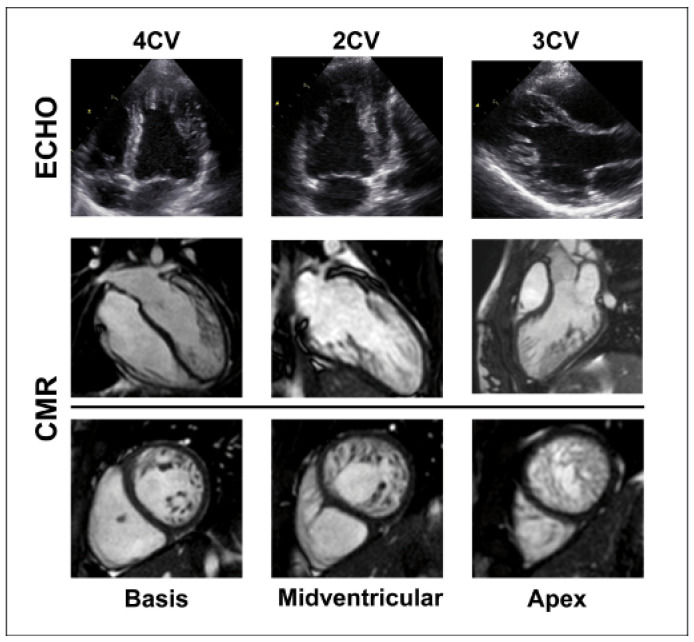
Echocardiography and cardiovascular magnetic resonance (CMR) imaging in left ventricular noncompaction (LVNC) of a 14-year-old girl with LVNC. The upper and middle panel show 4, 2, and 3 chamber views (CV) of representative echocardiographic and CMR images, respectively. The lower panel depicts the CMR short axis view at the basis, midventricular site, and apex of the same heart.
